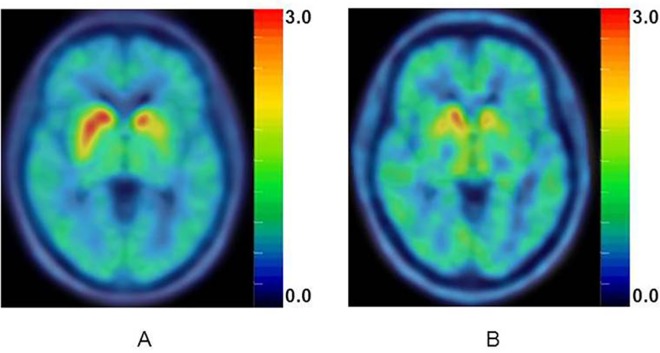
Representative ^18^F-AV133 SUVR images of PD patients with different CSF tau levels. A: a 60.7-y-old female with t-tau/p-tau of 23.8/6.5 pg/mL. B: a 58.9-y-old male with t-tau/p-tau of 29.8/13.9 pg/mL. The motor scale/MoCA score for the patient A and B were 10/30 and 35/25, respectively. ^18^F-AV133 ROI SUVRs of patient A and B were: caudate, 1.86 and 1.79; left putamen, 1.74 and 1.30; right putamen, 2.36 and 1.61; ventral striatum, 2.39 and 2.11; raphe nuclei, 1.50 and 1.73; and substantia nigra, 1.48 and 1.42, respectively.
